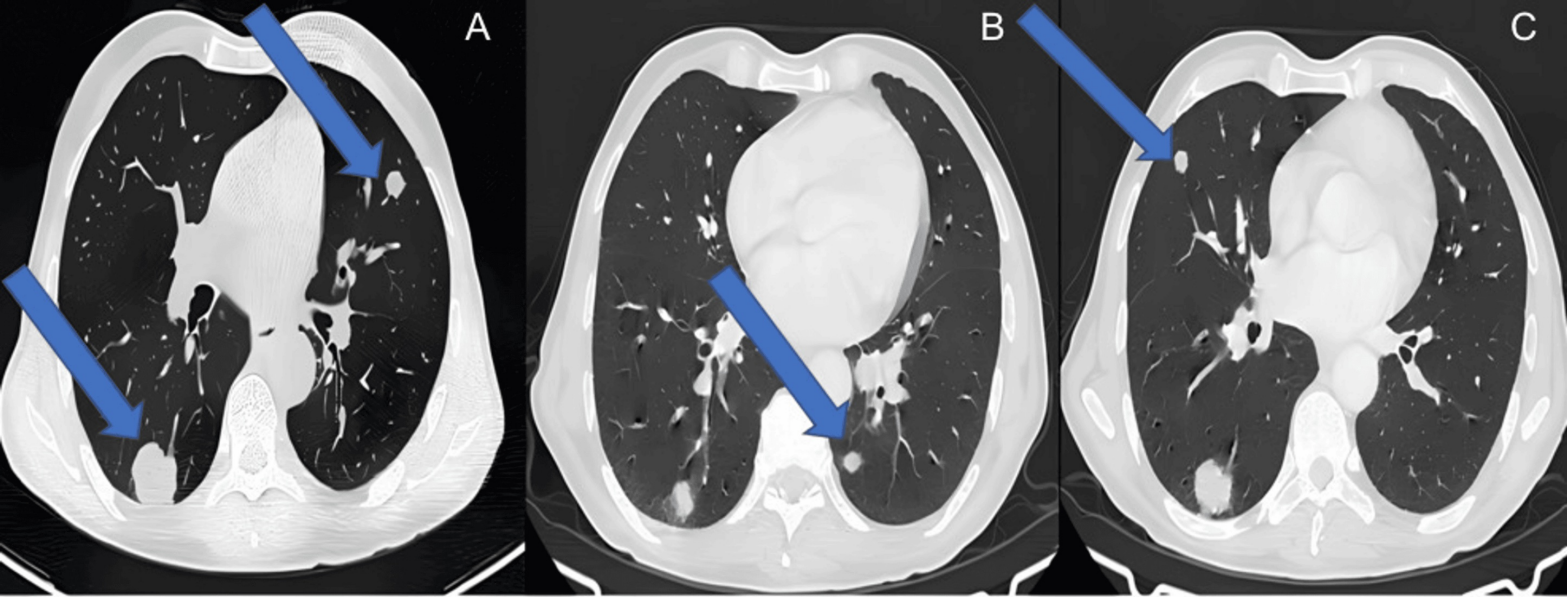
Axial slices of a contrast-enhanced chest CT scan in lung window A, B, C: axial slices of a contrast-enhanced chest CT scan in lung window, demonstrating multiple bilateral pulmonary nodules suggestive of secondary lesions (arrows). The largest nodule, located in the right lower lobe and measuring 30 × 28 mm, is shown in A.
